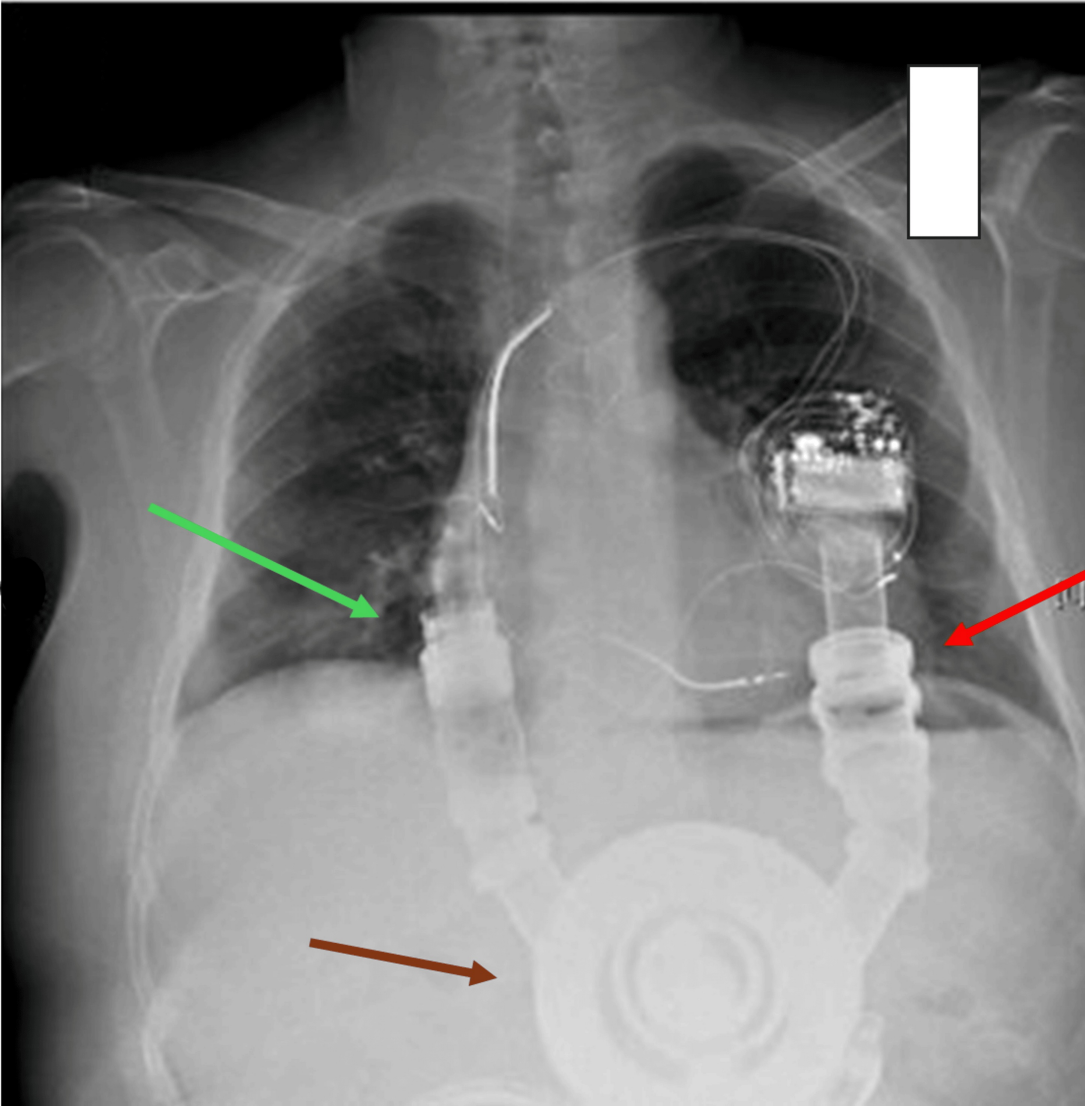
The X-ray shows a patient with a first-generation HeartMate 1 LVAD implant; it is larger than the third-generation model. The drive line is not visible on the X-ray, which was connected to an external controller; this controller connected the external batteries. Red arrow: inflow-valve housing; green arrow: outflow-valve housing; brown arrow: prosthetic left ventricle. This image was provided by the author (Bordoni Bruno) of this article. LVAD: left ventricular assist device HeartMate 1 (HM1; Chicago, IL: Abbott)
